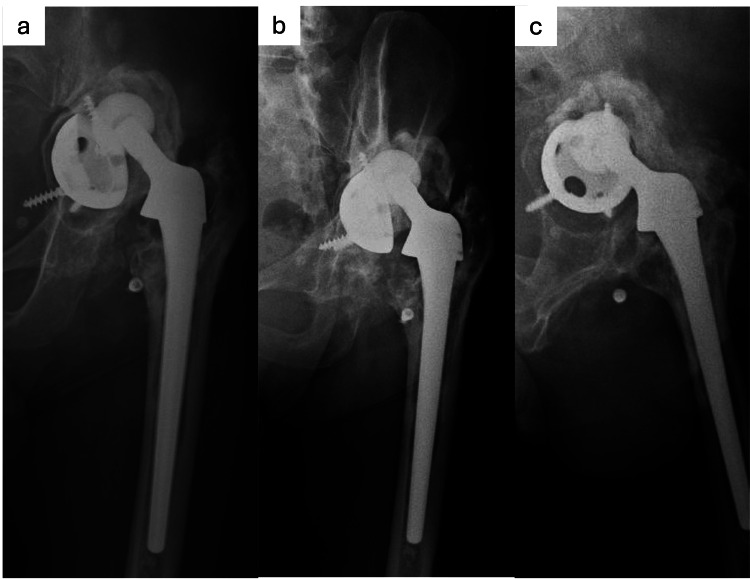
Hip radiographs a, b, c: In different projections obtained at 15-year follow-up after Lord THA (revision total hip arthroplasty), showing aseptic loosening of the acetabular cup with massive acetabular bone loss and stability of the Lord femoral stem.
